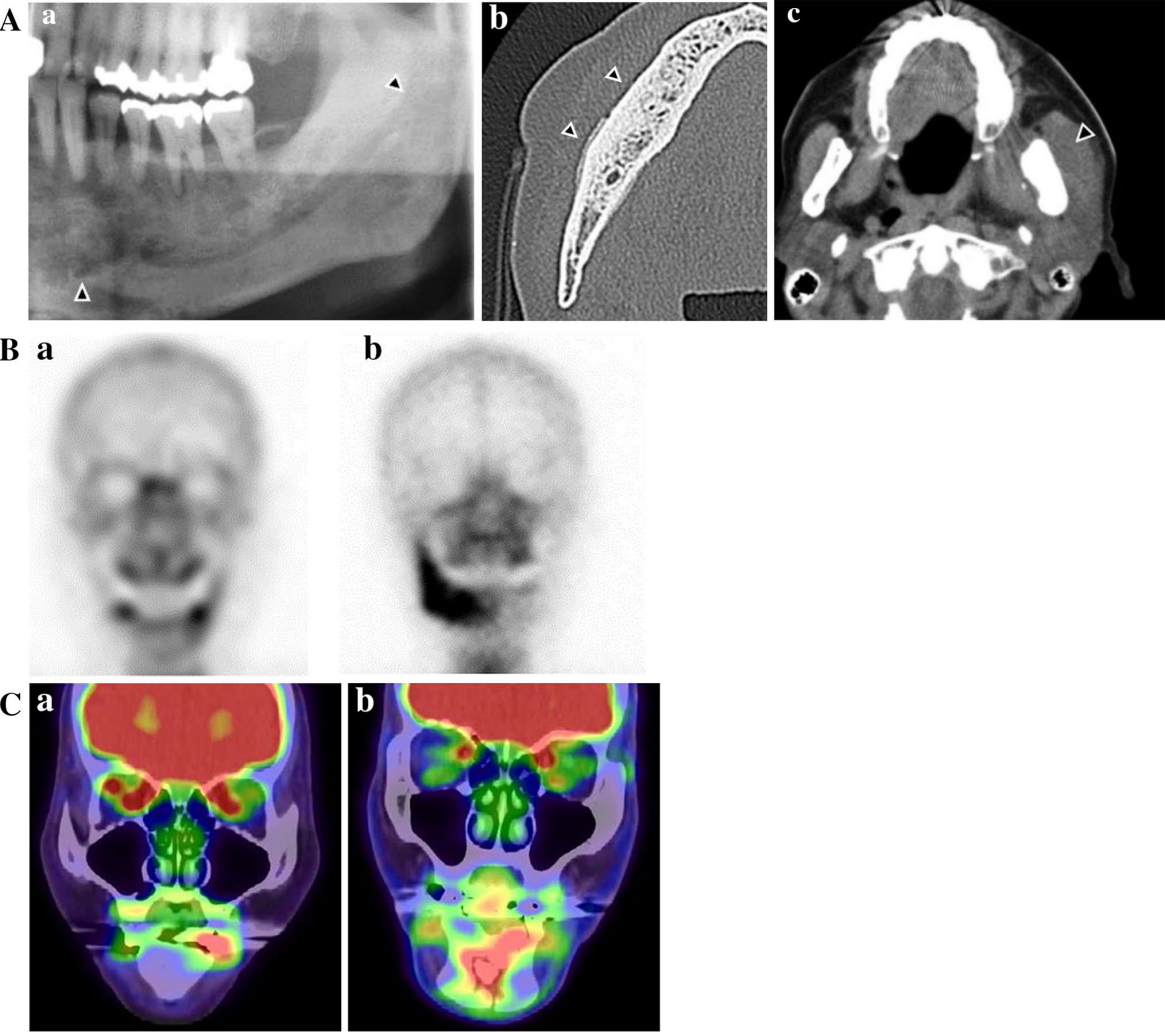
Grading of imaging features. A Imaging features of DPR and CT in grading criteria. a DPR showing osteosclerotic changes extending into the mandibular canal (black arrow). DPR score 1: Grade 2. b CT findings showing a periosteal reaction (black arrow). CT score 2: Grade 1. c CT findings indicating the spread of inflammation from the jaw to the masticatory muscles (white arrow). CT score 3: Grade 2. B Imaging features of BS in grading criteria. a Spot accumulation pattern. BS score: Grade 1. b Spread pattern. BS score: Grade 2. C Imaging features of FDG-PET/CT in grading criteria. a Spot pattern; PET score: Grade 1. b Spread pattern; PET score: Grade 2. *BS* bone scintigraphy, *DPR* dental panoramic radiography, *CT* computed tomography, *FDG* 2-(18F)-fluoro-2-deoxy-D-glucose, *PET* position emission tomography
